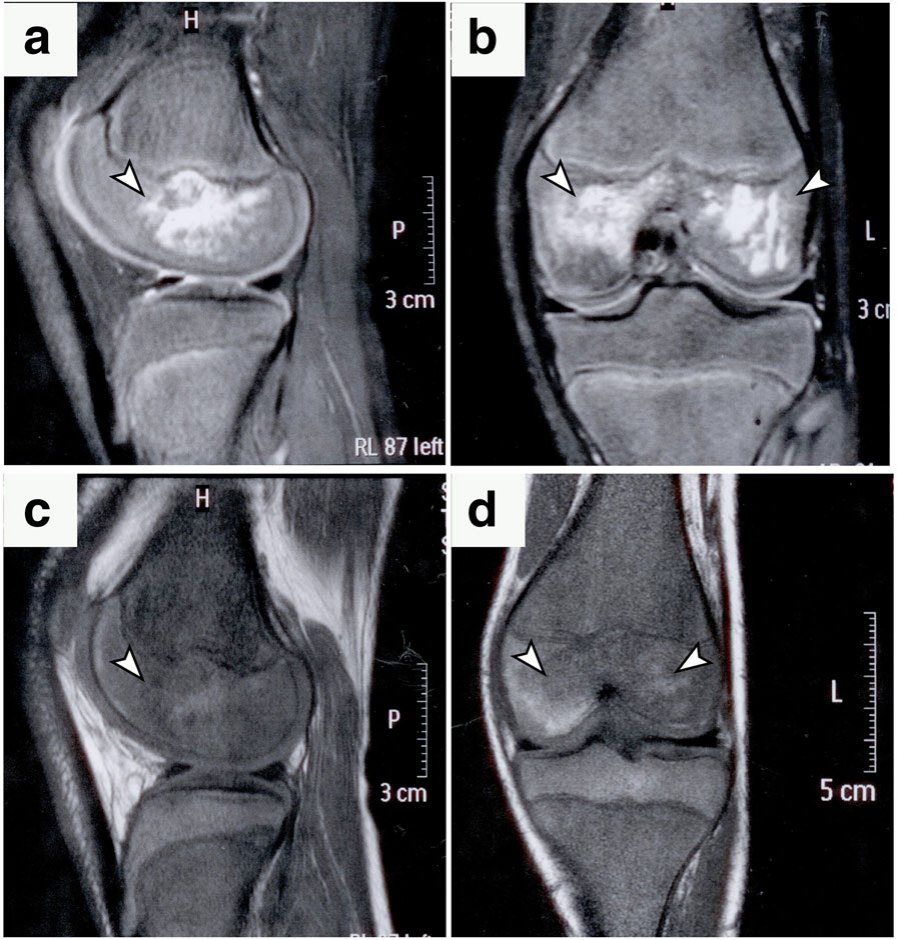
MR imaging after successfully treatment with BMC. a - c MR imaging of the 16-years-old boy before (a, b) and after surgery (c, d, 12 months postoperatively). PD-weighted (a, b) and T1-weighted (c, d) sequences demonstrate no subchondral bone erosion or fracture, normal contour of the epiphyseal head and preservation of the joint space. Proton density-weighted (a, b) sequences demonstrate mostly hematopoietic marrow without cystic or fibrotic lesion
